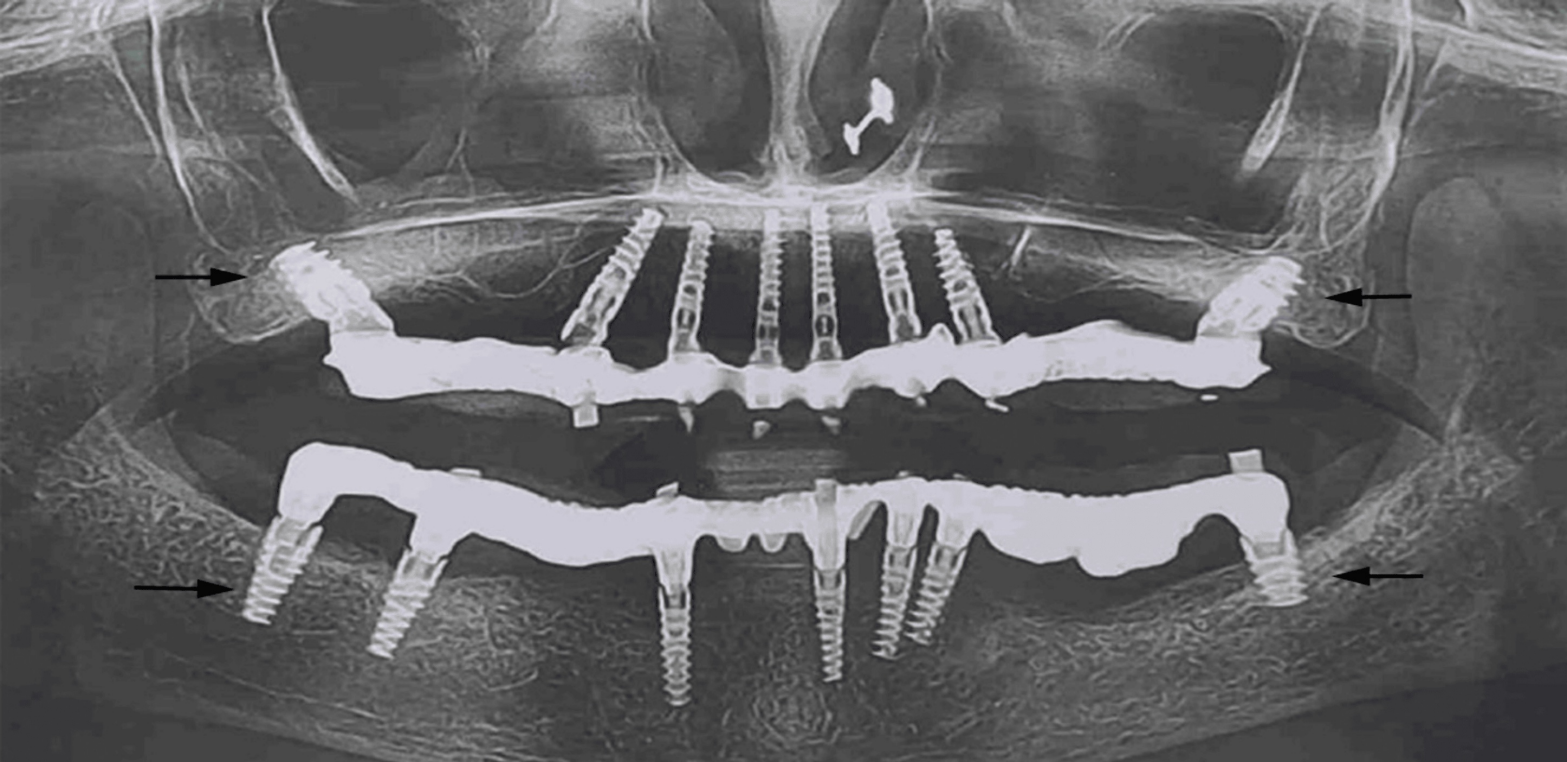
Post-rehabilitation orthopantomogram.
